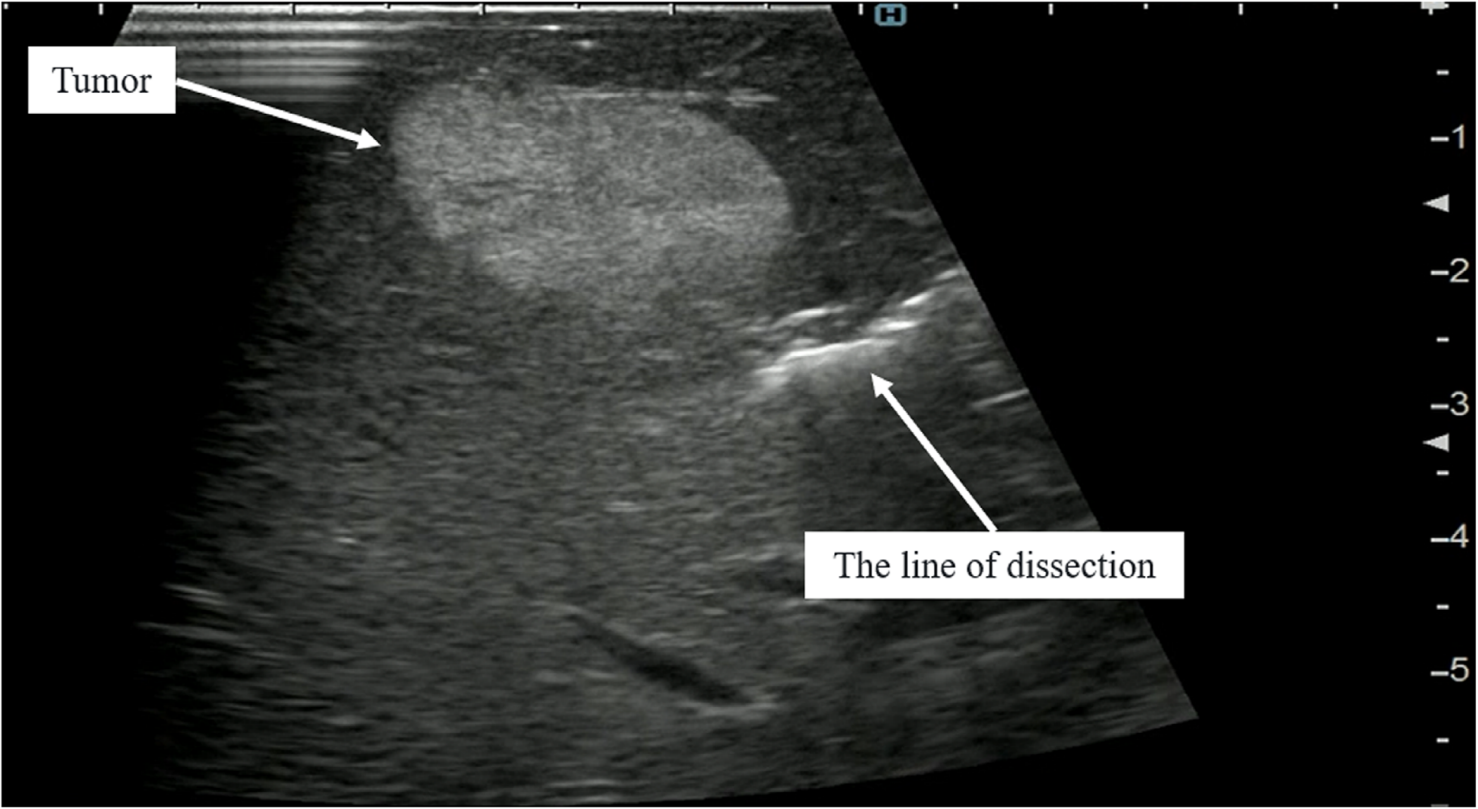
An image of intraoperative ultrasound during liver dissection is shown. Intraoperative ultrasound was performed as needed to assess the relationship between the tumor and the line of dissection and to confirm whether the deepest tumor margin had been secured.
